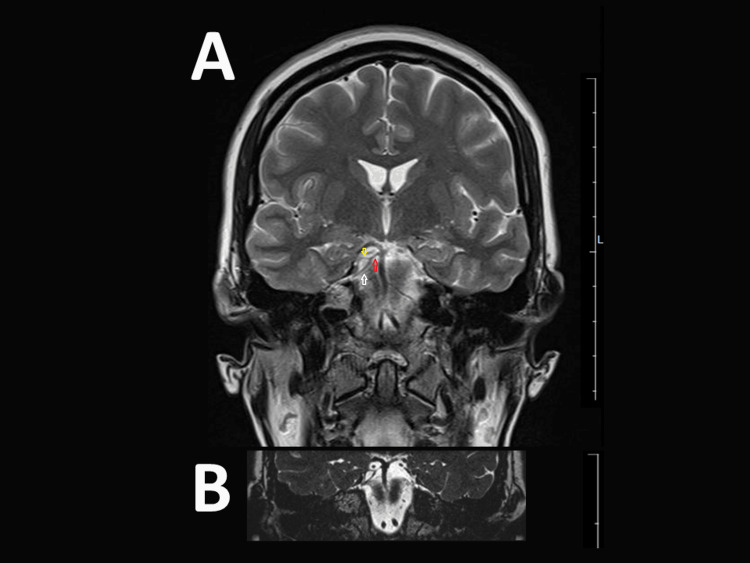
Coronal magnetic resonance imaging (MRI) Coronal view of an MRI T2 weighted image showing bifurcation of the right superior cerebelar artery (2A and 2B): Red arrow: superior cerebellar artery before bifurcation; Yellow arrow: superior branch of superior cerebellar artery; white arrow: inferior branch of superior cerebellar artery.
